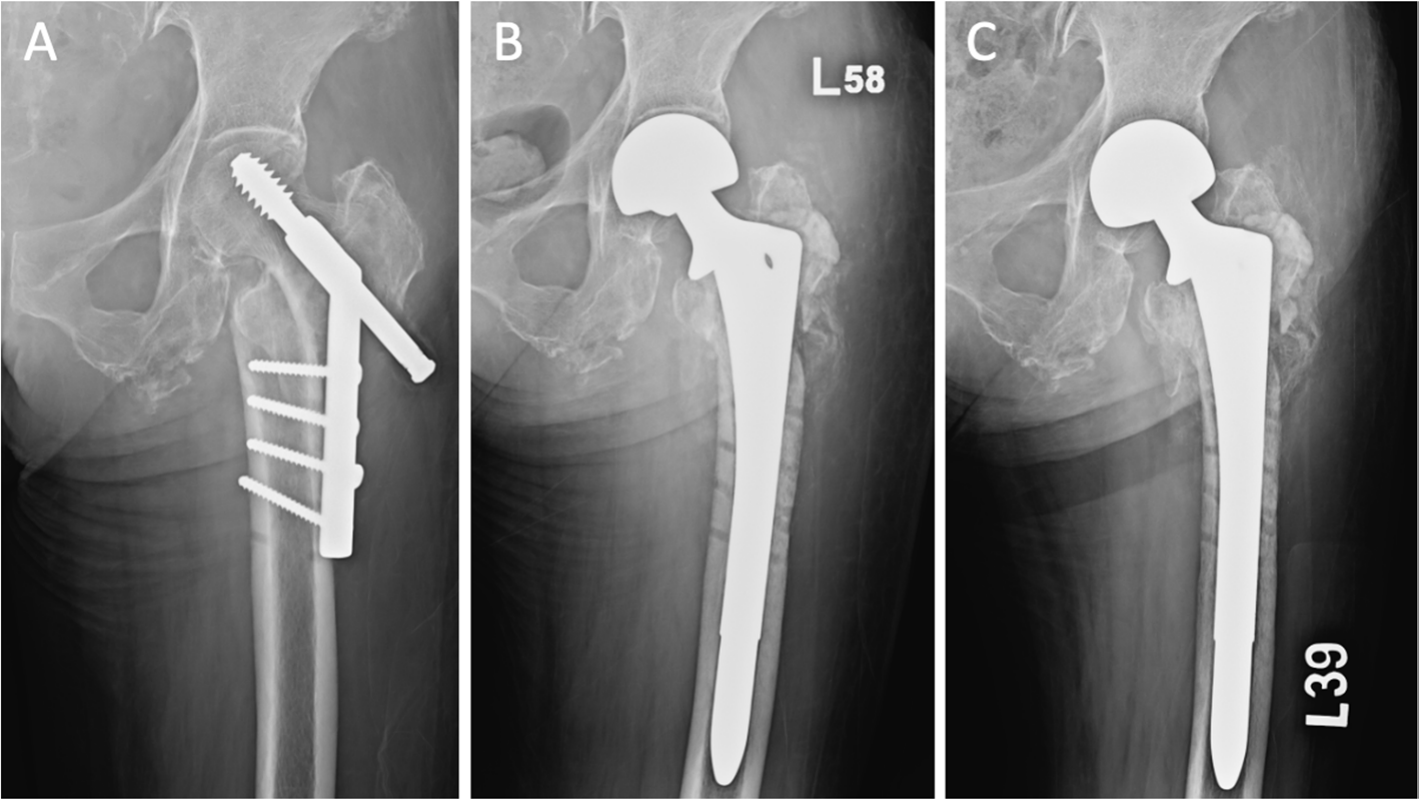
(A) 72-year-old female, with early fixation failure (postoperative 4 weeks, fracture collapse), (B) bipolar hemiarthroplasty with cementless revision stem, immediate postoperative radiograph, (C) postoperative 22 months
